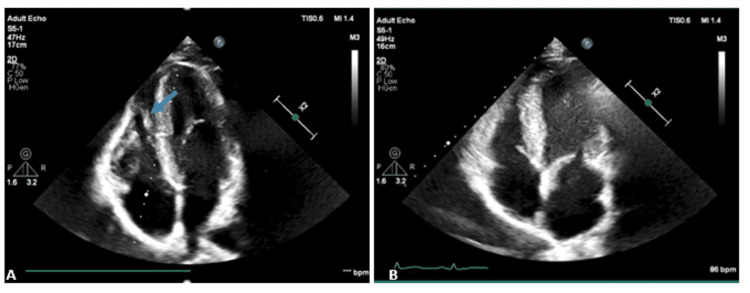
Transthoracic echocardiogram in the acute phase and after six months 2A. Transthoracic echocardiogram in four-chamber view revealing an oval-shaped mass (blue arrow) in the apex of the right ventricle 2B. Follow-up transthoracic echocardiogram after six months of adequate anticoagulation showing complete resolution of the thrombus
